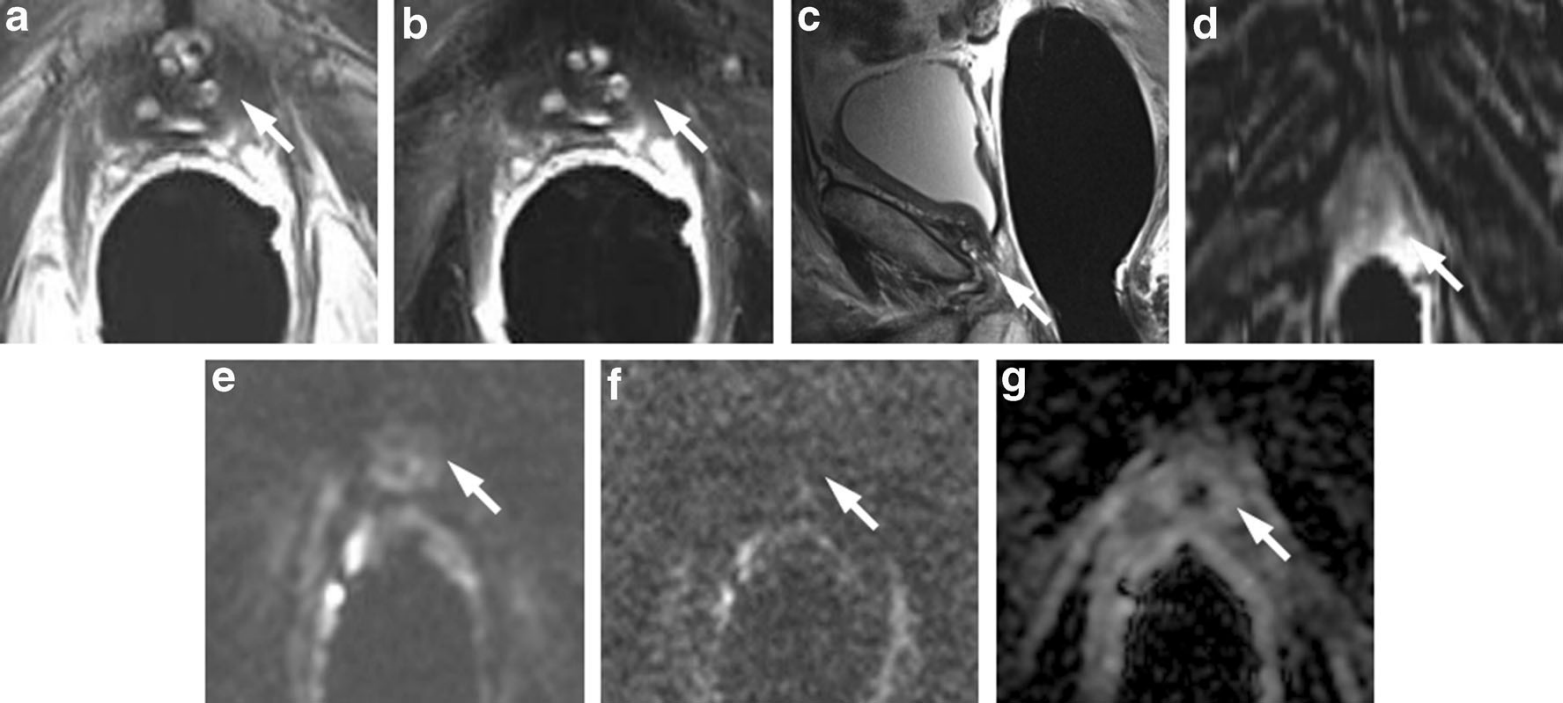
MR images of a 61-year-old man with prostate-specific antigen progression (PSA serum level 0.62 ng/mL) after radical retropubic prostatectomy, with suspected local recurrence. a Axial T2-weighted fast spin-echo (5170/112), b axial T2-weighted fat saturated fast spin-echo (6920/112) and c sagittal T2-weighted fast spin-echo (4730/114) image showing a hyperintense lobulated tissue on anterior perianastomotic location posterior to the pubic symphysis d Axial perfusion Gradient-echo T1-weighted subtracted image showing no signs of enhancement of the suspicious pathological tissue. e Diffusion-weighted images with a b value of 1.000, *f* 3.000 s/mm^2^ and *g* ADC map show no diffusion restricted phenomena. The hyperintese lobulated tissue detected in T2-weighted images represents a prominent sealed off venous plexus (*white arrows*)
